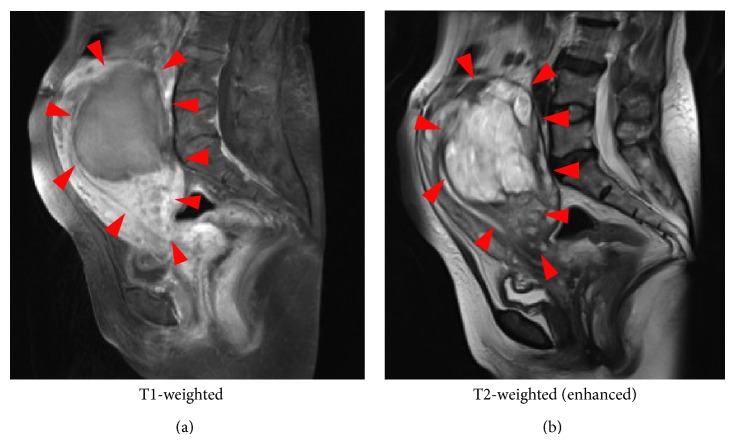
Frontal pelvic magnetic resonance imaging demonstrated a lobular tumor with low T1- and high T2-signal intensity on the posterior wall of the uterus.
